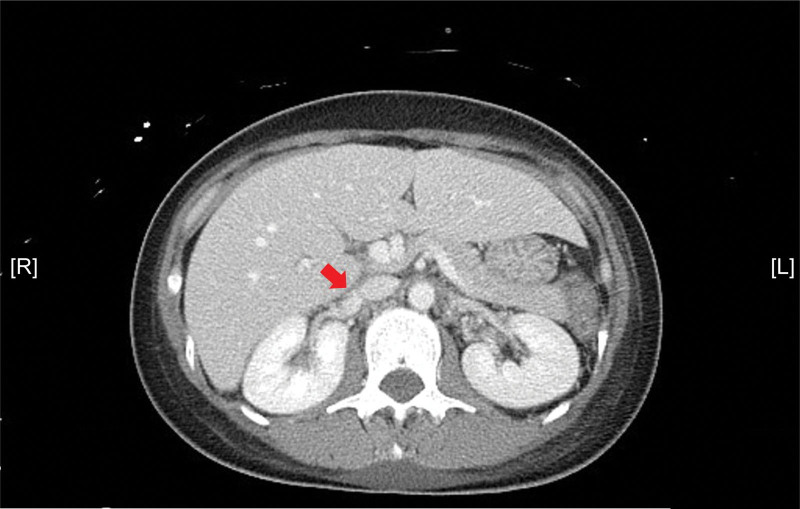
Contrasted abdominal CT images revealing improvement with approximately 90% dissolution of thrombus within the right renal vein. CT = computed tomography.
